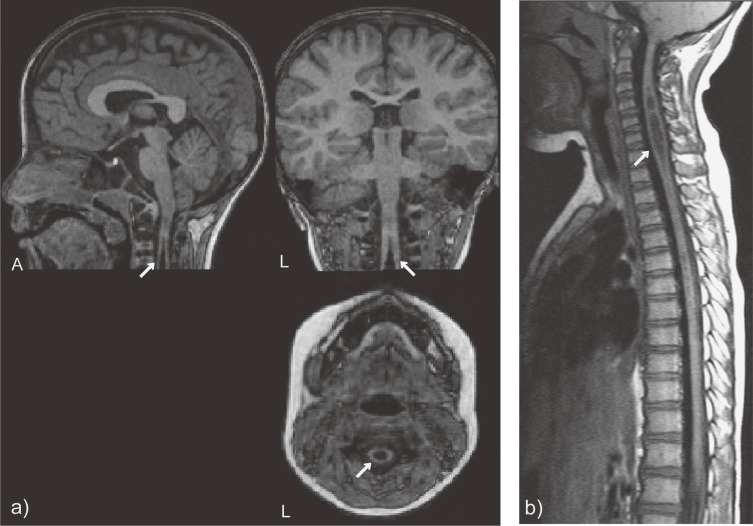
A incidental finding classified into routine referral category: Cervical syringomyelia. a) 3D-T1 weighted images obtained in our research, b) Spinal MRI acquired as further examination after referral. Arrows indicate the lesions. (A: anterior, L: left)
